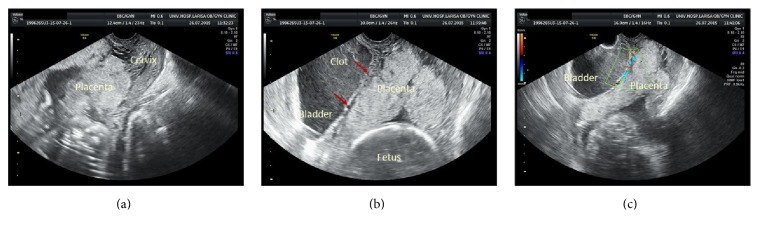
(a) Complete placenta previa. (b) Disruption of the bladder-placental interface indicated by red arrows. (c) Color Doppler revealed hypervascularity between the placenta and the bladder.
